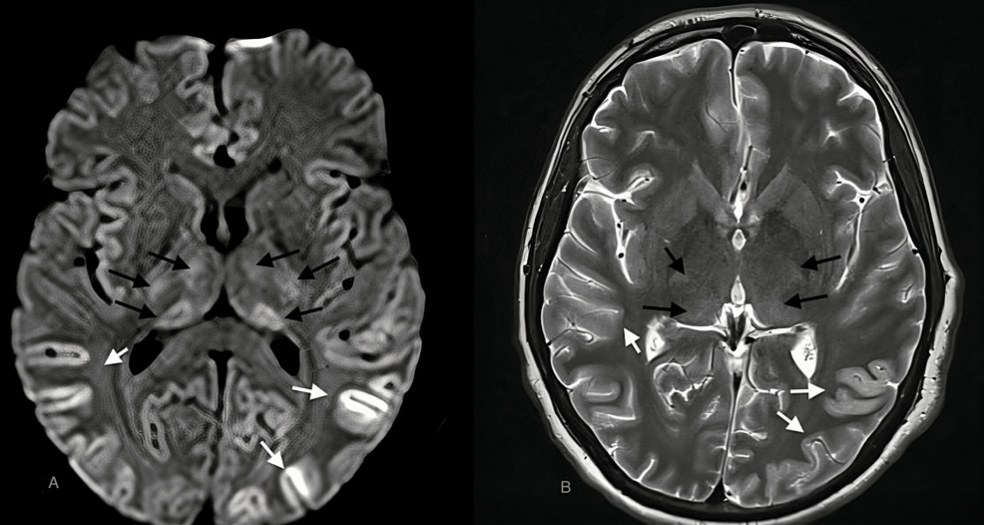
Case 5: New interval increase in hyperintense areas in DWI and T2W images are identified in both parieto-occipital lobes and thalami of a 40-year-old male patient six days after presentation with dengue encephalitis (white arrows and black arrows, in A and B, respectively). DWI: diffusion-weighted image; T2W: T2-weighted image
